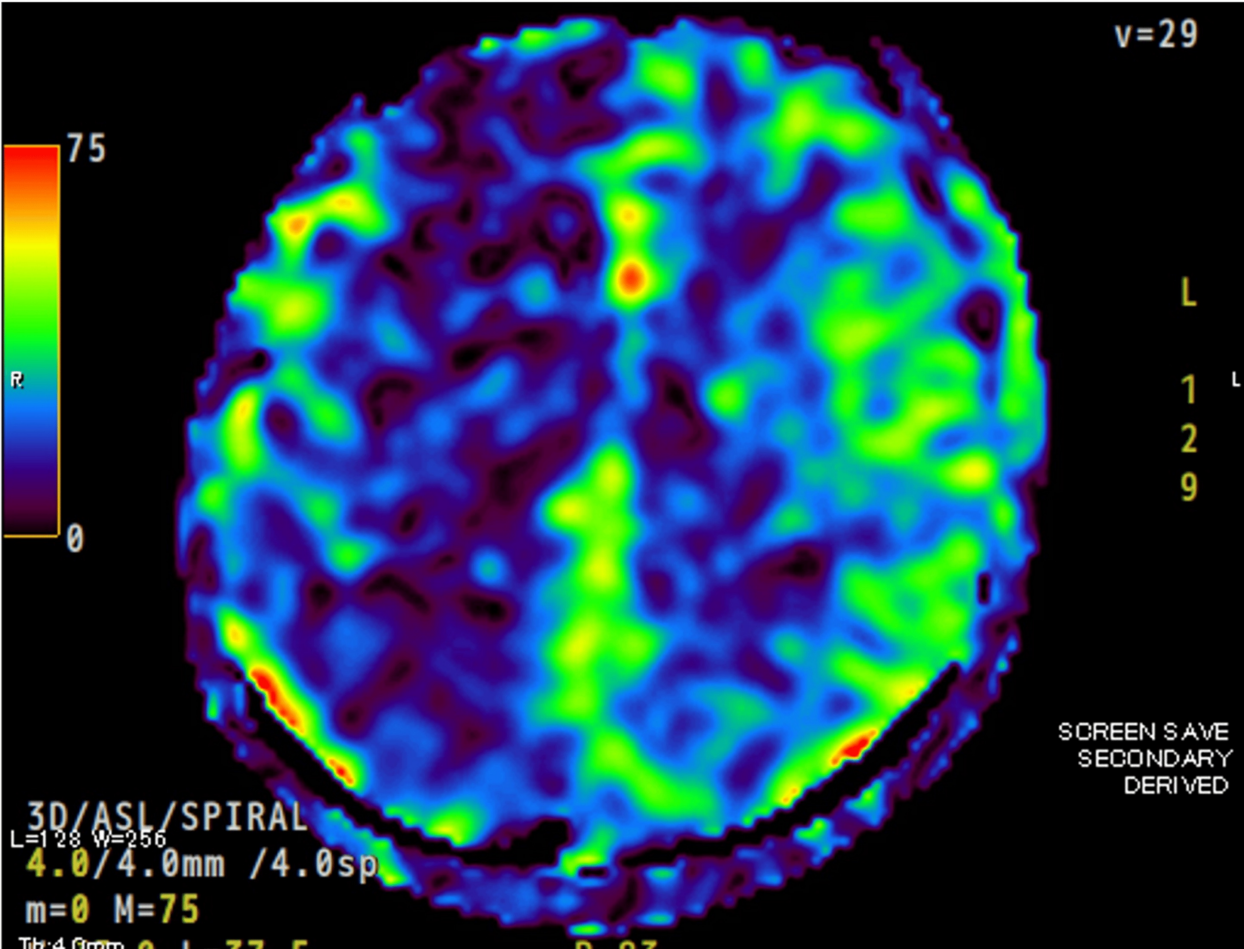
Brain ASL-MRI showing hypoperfusion in the right hemisphere. MRI: magnetic resonance imaging; ASL-MRI: arterial spin labeling MRI.
